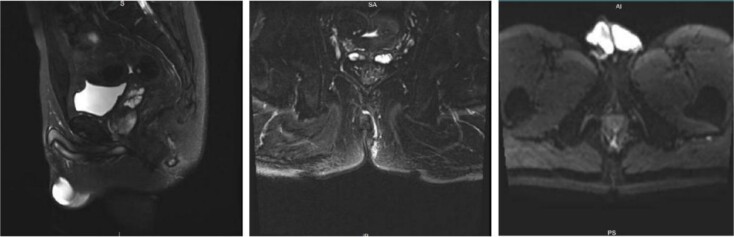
Preoperative perianal magnetic resonance imaging (MRI) examination.
